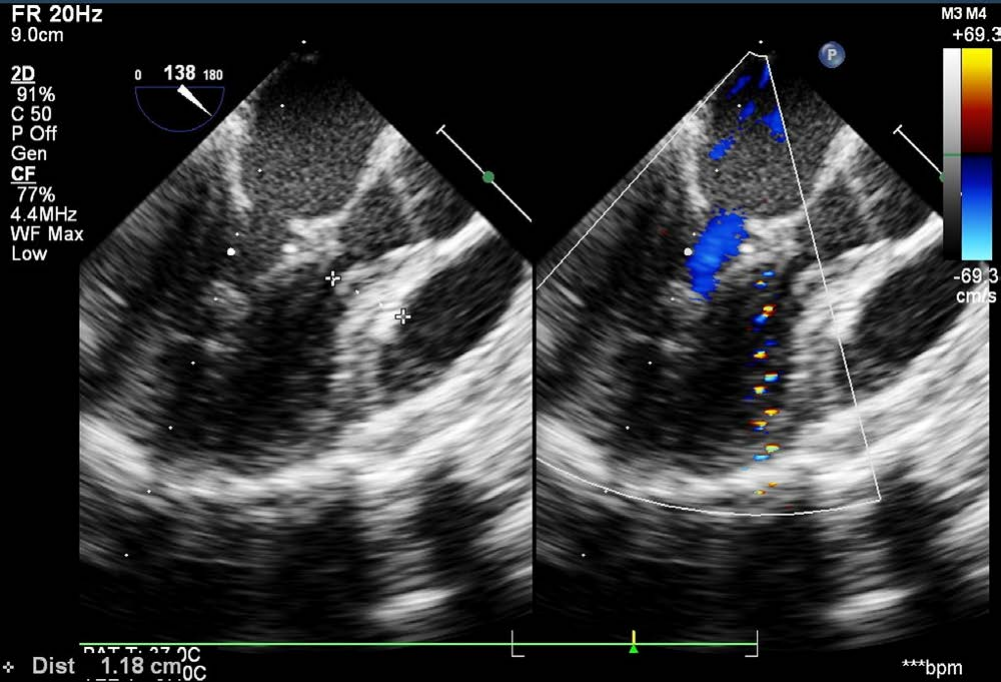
Cardiac ultrasound before surgery.
